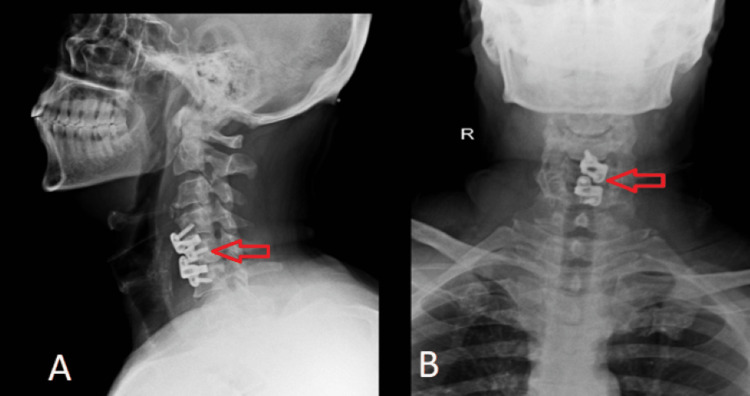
Postoperative X-ray of the cervical spine (A) Lateral view of X-ray where arrows indicate the placement of Jayon spacer between C4-C5 and C5-C6 level. (B) Anteroposterior view of the X-ray; red arrows indicate the spacer at multiple levels
